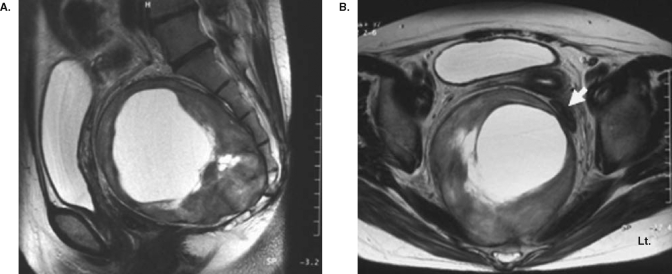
Sagittal image (A) and axial image (B) of T2-weighted MRI showing a giant tumor with a cystic component at the presacral retroperitoneal space displacing intrapelvic organs including the bladder and rectum (white arrow). To excise the tumor, the resection and re-anastomosis of the sigmoid colon was performed by the anterior approach.
